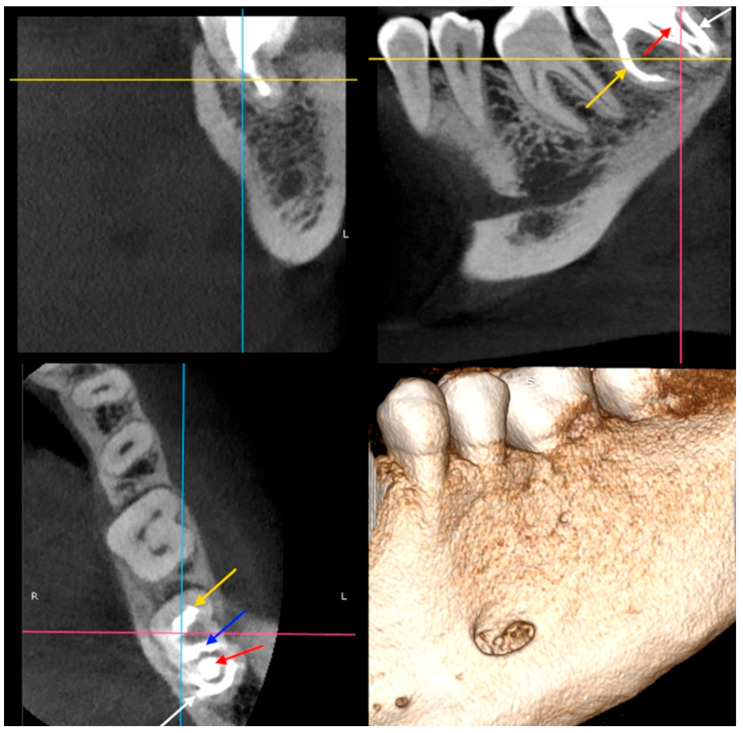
Tooth 3.7—CBCT scans 9 months post-operative: yellow arrow—mesial canals, blue arrow—two distal canals, white arrow—semicircular space, red arrow—the additional tooth.
